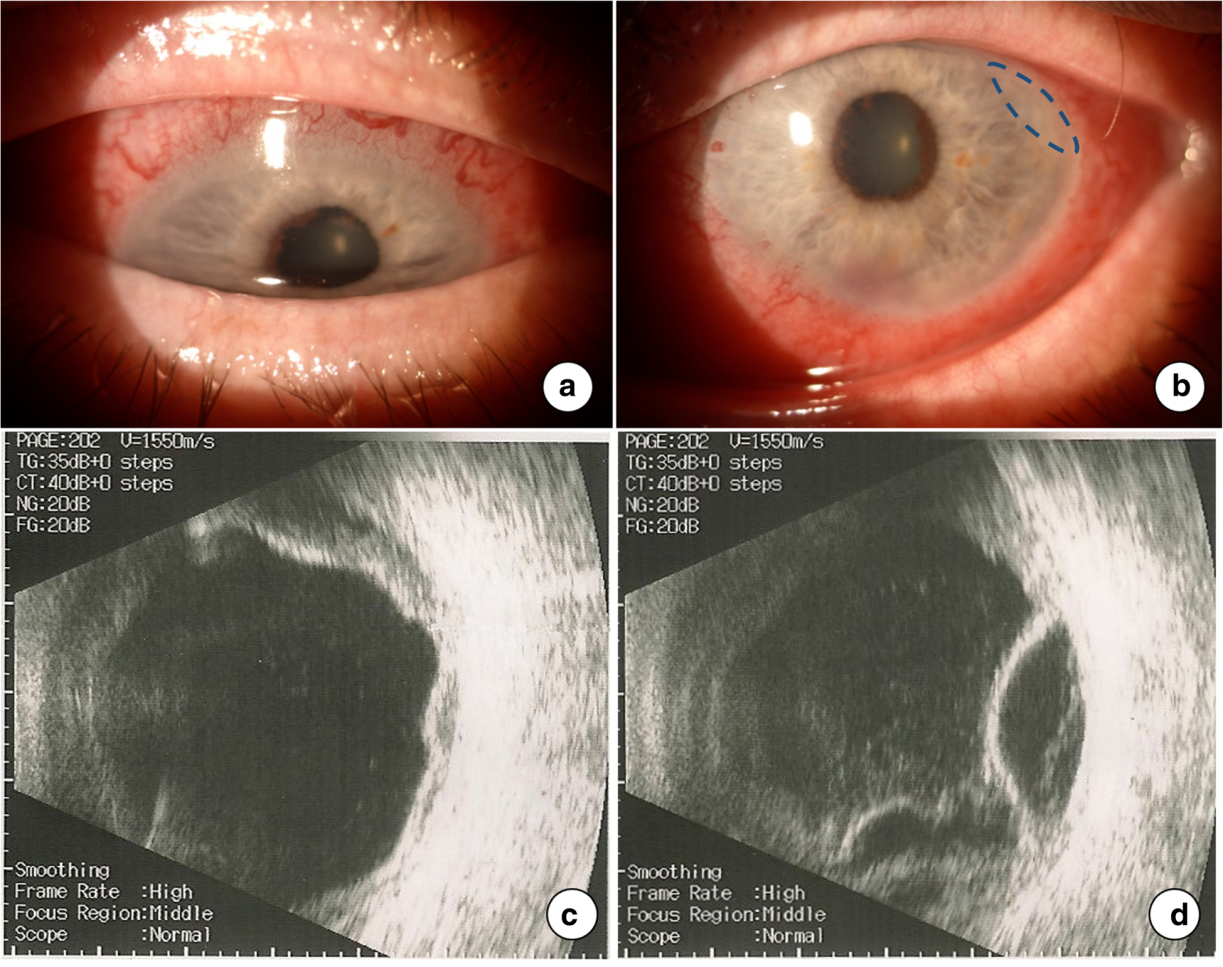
Examination at presentation. Diffuse scleritis, mild paralimbal keratitis and posterior synechiae in the right (a) and left eye (b). Ultrasound examination showed inflammation of the vitreous body and exudative retinal detachment in the right (c) and left eye (d). The blue dashed line delineates the location of keratitis
